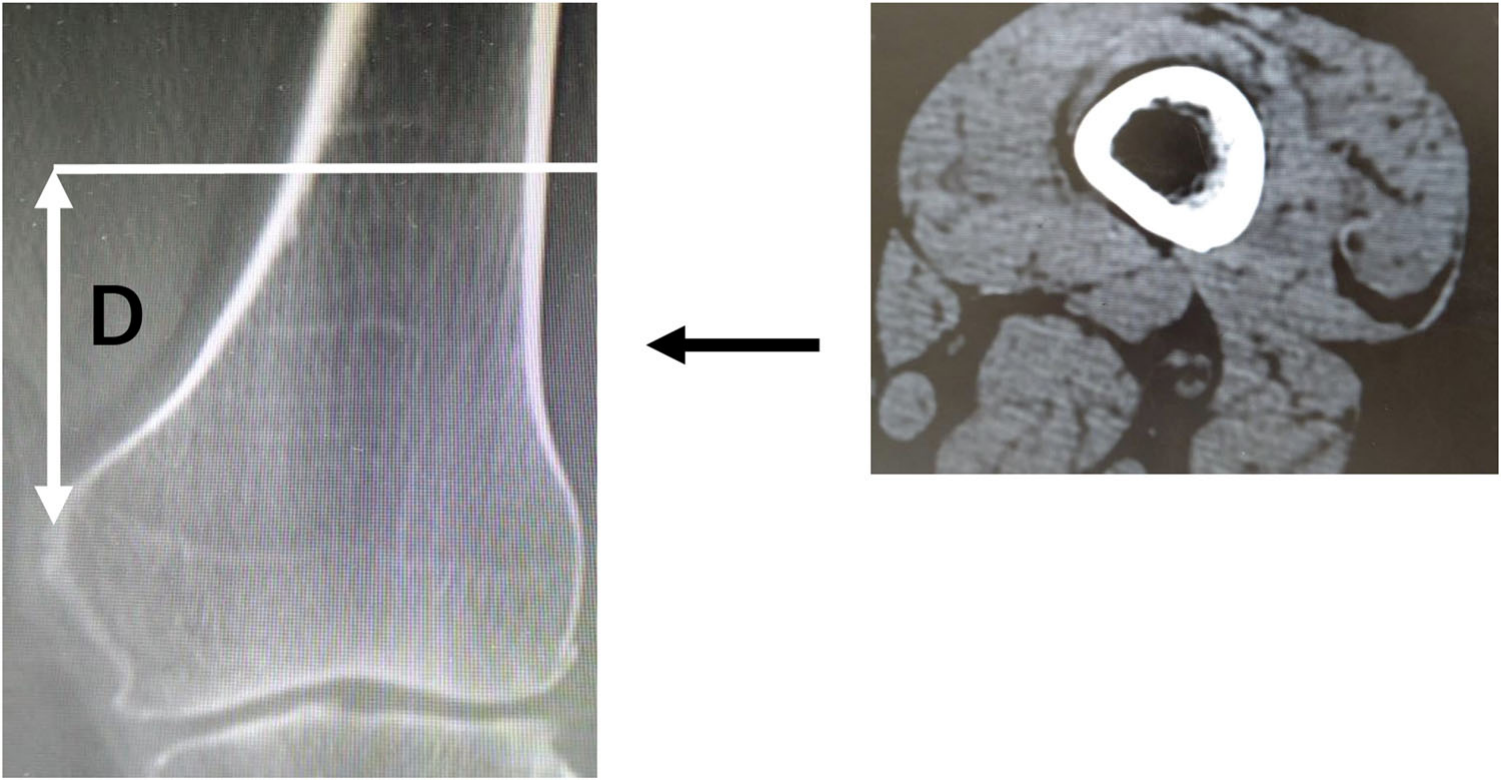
Cross‐sectional plain computed tomography (CT) images for measurement. Full‐length plain CT image of the lower extremity in supine position, with the patella facing forward, 0.65 mm thick, and perpendicular to the functional axis. The first cross‐section on CT where the vastus medialis muscle and popliteal artery distally connect to the adductor hiatus was used.
